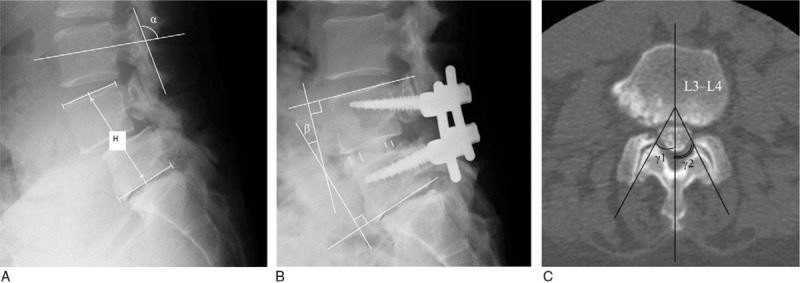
Measurement methods of the parameters on plain radiographs and computed tomography scans. (A) The L4-L5 vertebral height (H) and L3 lamina inclination (α). The L3 lamina inclination was defined as the angle formed by a line connecting the base of the superior facet joint and that of the inferior facet joint, and a horizontal line bisecting the vertebral body. (B) The L4-L5 fusion angle (β) was measured using the Cobb method. (C) γ1 is the right facet angle and γ2 is the left facet angle. Facet sagittalization and tropism were defined as (γ1 + γ2) and (γ1 − γ2), respectively.
